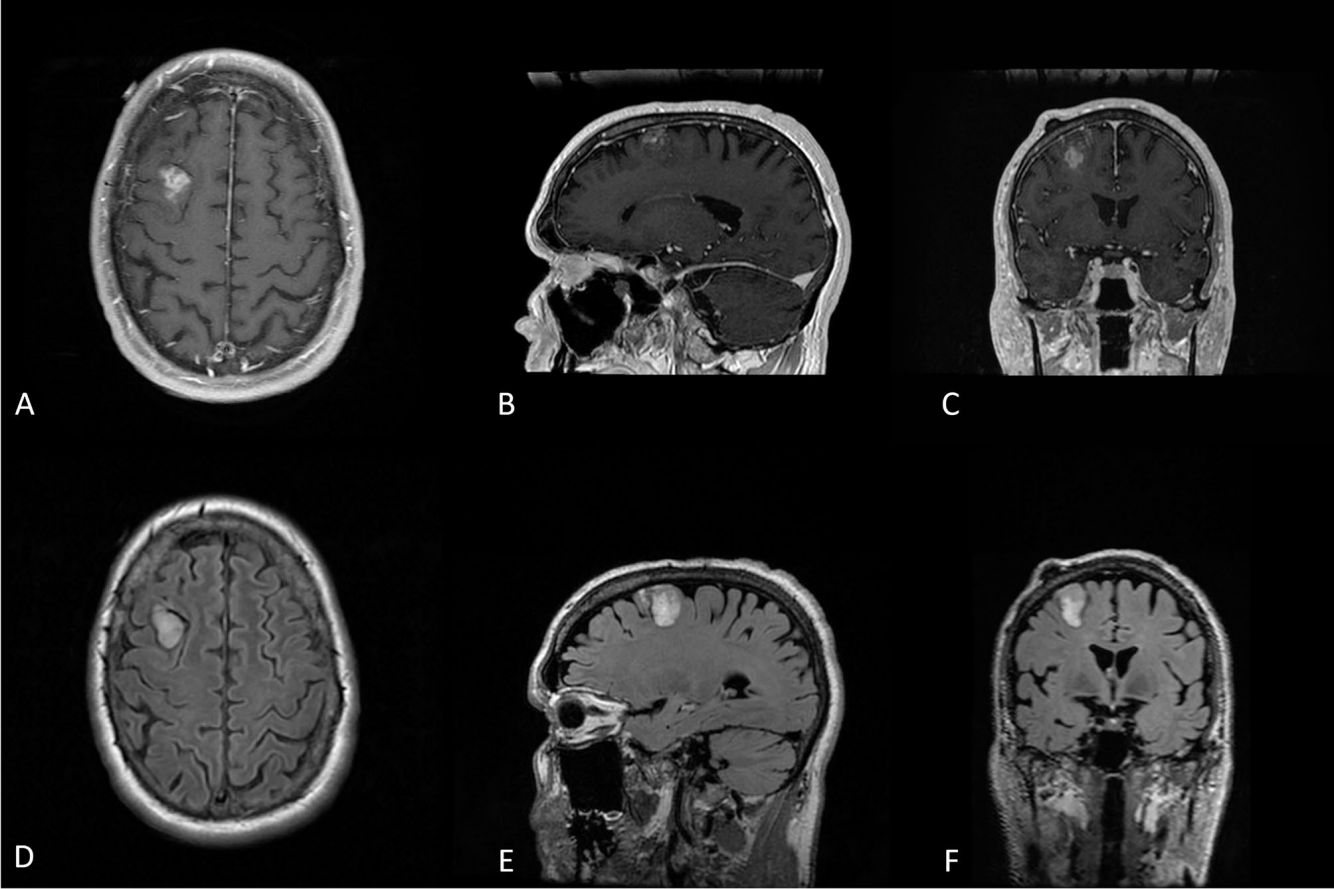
Axial (A), sagittal (B), and coronal (C) cuts of a T1-weighted post-contrast MRI and axial (D), sagittal (E), and coronal (F) cuts of a T2 FLAIR MRI brain in a 62 year old man with an incidentally discovered mass in the right middle frontal gyrus. The post-contrast images in A-C demonstrate serpiginous enhancement. D-F highlight FLAIR hyperintensity without surrounding vasogenic edema.An unrelated osteoma can also be seen overlying the mass
